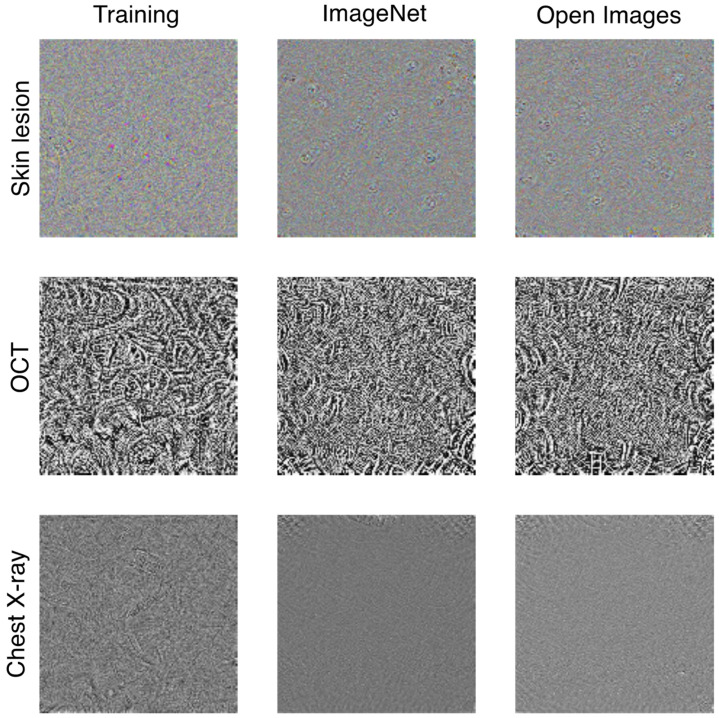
Visualization of non-targeted UAPs generated using training (medical image), ImageNet, and Open Images datasets against Inception V3 models for skin lesion, OCT, and chest X-ray image classifications. UAPs are visually emphasized for clarity; specifically, each UAP is scaled by a maximum of 1 and minimum of zero.
